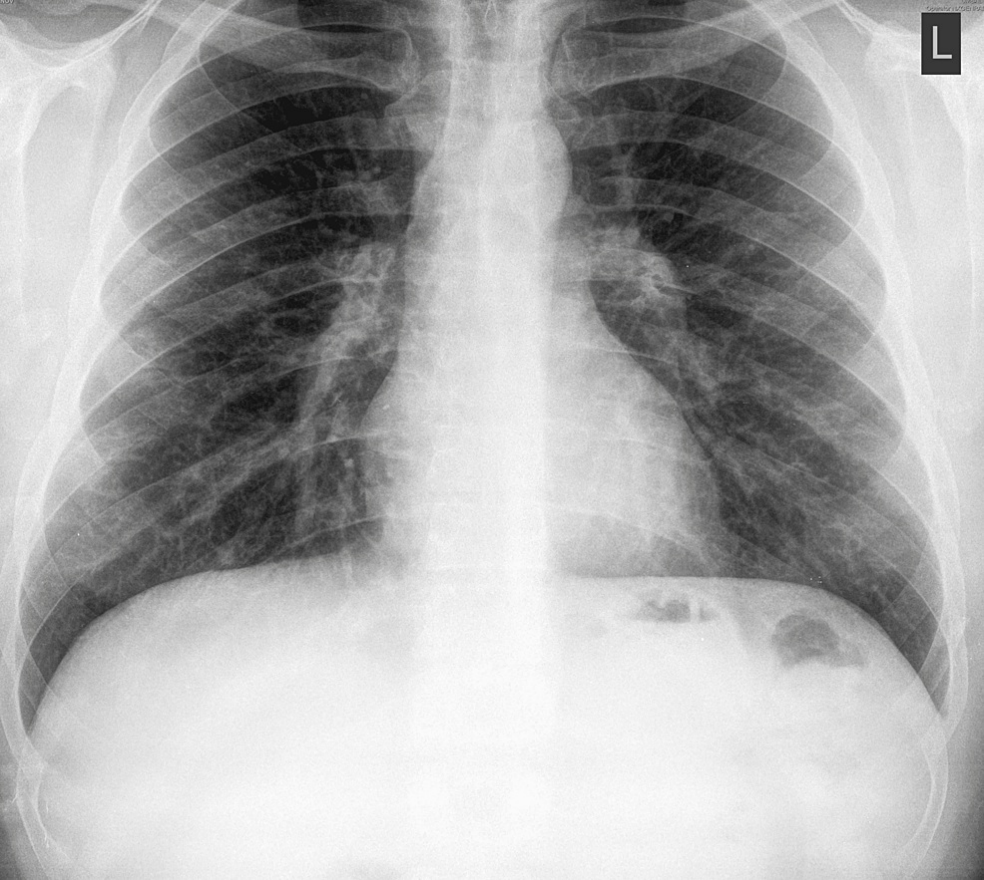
Chest X-ray of the patient upon hospital admission The image demonstrates fine peripheral areas of ground glass opacities with central reticular interstitial thickening.
